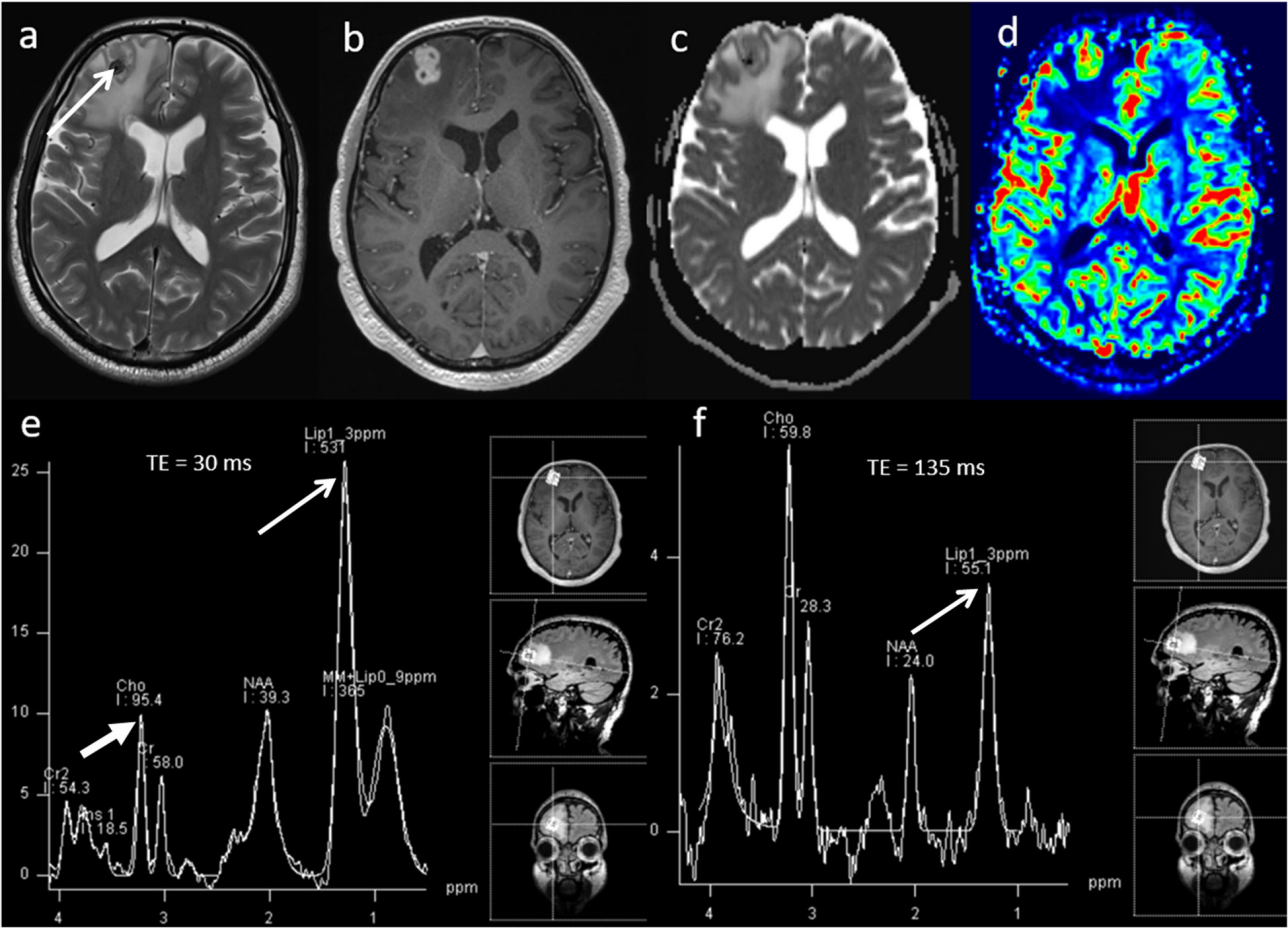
Tuberculoma. Conventional MRI Findings: a, b Axial T2W and post-contrast T1W sequences show T2W hypointense confluent lesions in the right frontal lobe with extensive perilesional oedema and enhancement. Multiparametric MRI: c ADC map shows intermediate values (900 × 10^−6^ mm^2^ s^−1^), (d) PWI shows perfusion higher than the contralateral white matter, (e, f) MRS shows very high levels of lipid at 1.3 ppm (thin arrows), without any lactate. There is slightly elevated Cho/Cr ratio (1.5) on short TE MRS (thick arrow), moderately low NAA/Cr ratio and absence of mI. In this case of tuberculoma, the combination of a T2W hypointense lesion, raised rCBV, raised lipids and moderately increased Cho/Cr ratio helped to make the diagnosis. The patient commenced anti-tuberculosis treatment, and surgical intervention was avoided.
